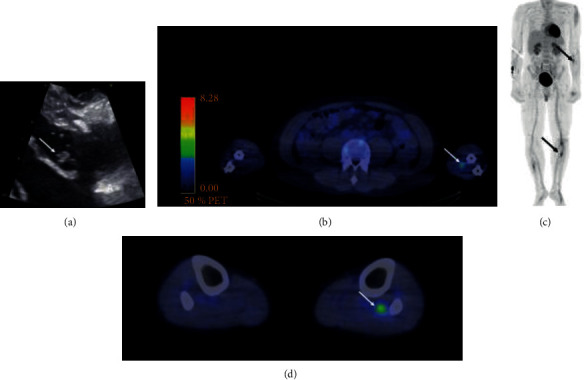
Transesophageal echocardiography images of patient 2 (panel (a)). Arrow shows vegetation. ^18^F-FDG-PET/CT images of patient 2 (panels (b)–(d)). Arrows show ^18^F-FDG uptake at the site brachial artery and fibular artery.
